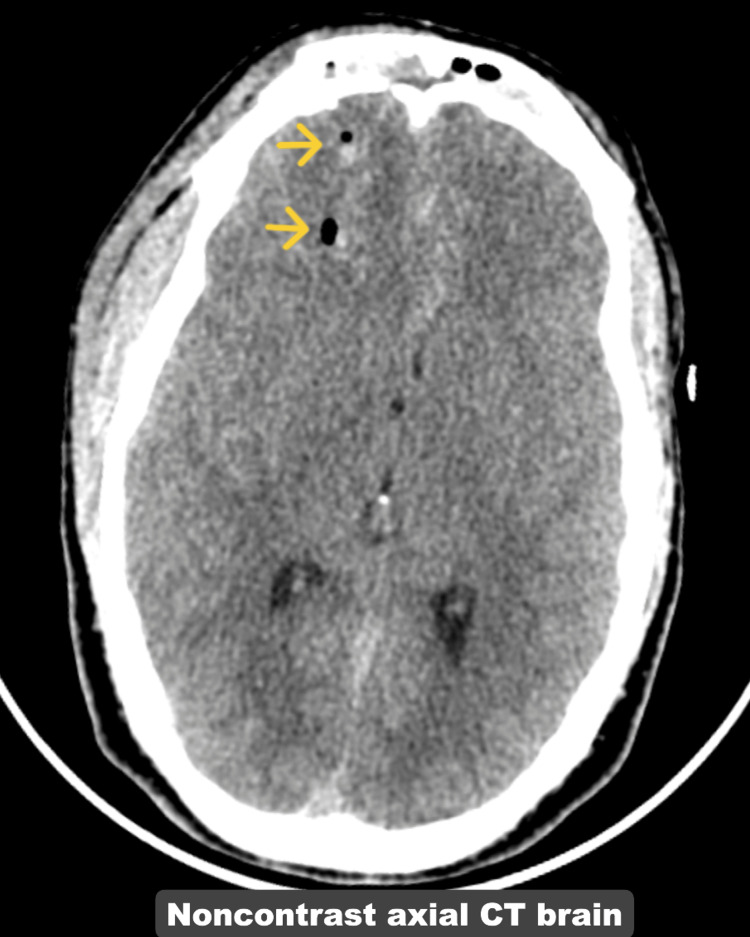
Day 0 (10 hours) non-contrast axial CT brain showing persistence of intracranial air foci Yellow arrows show persistent intracranial air foci (pneumocephalus) with a mild increase in the surrounding hemorrhagic contusions in the right frontal region.
